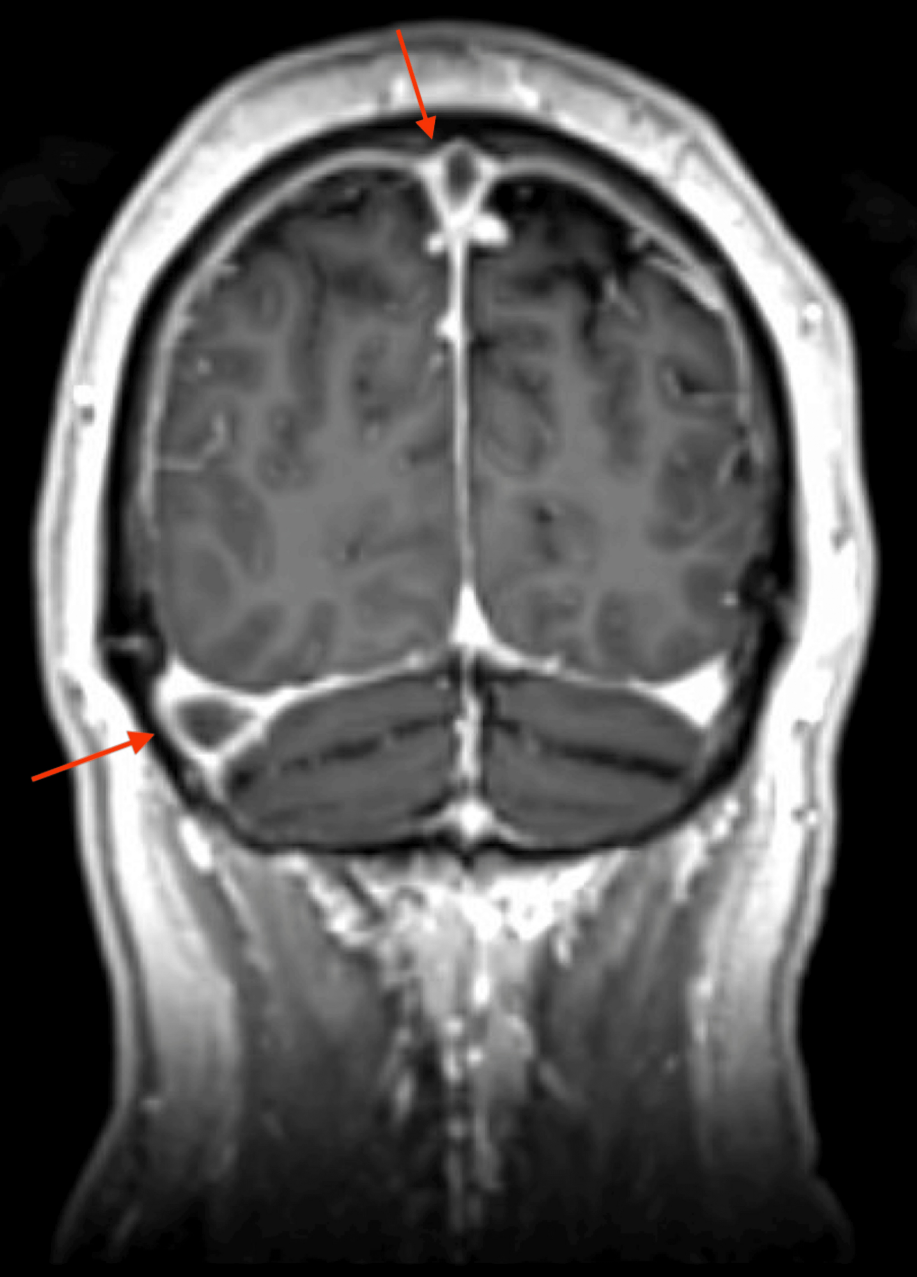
MRI of the brain with contrast, coronal view, demonstrating multisinus involvement including the right transverse sinus (arrow) and superior sagittal sinus (arrow)
